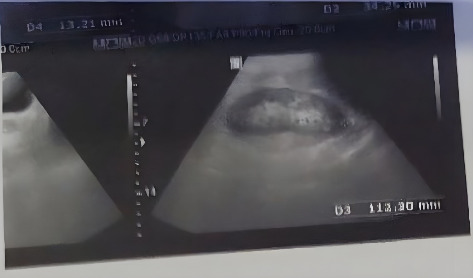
Abdominopelvic sonography showing a 13 mm echogenic mass (hemangioma) in the right lobe of the liver.
